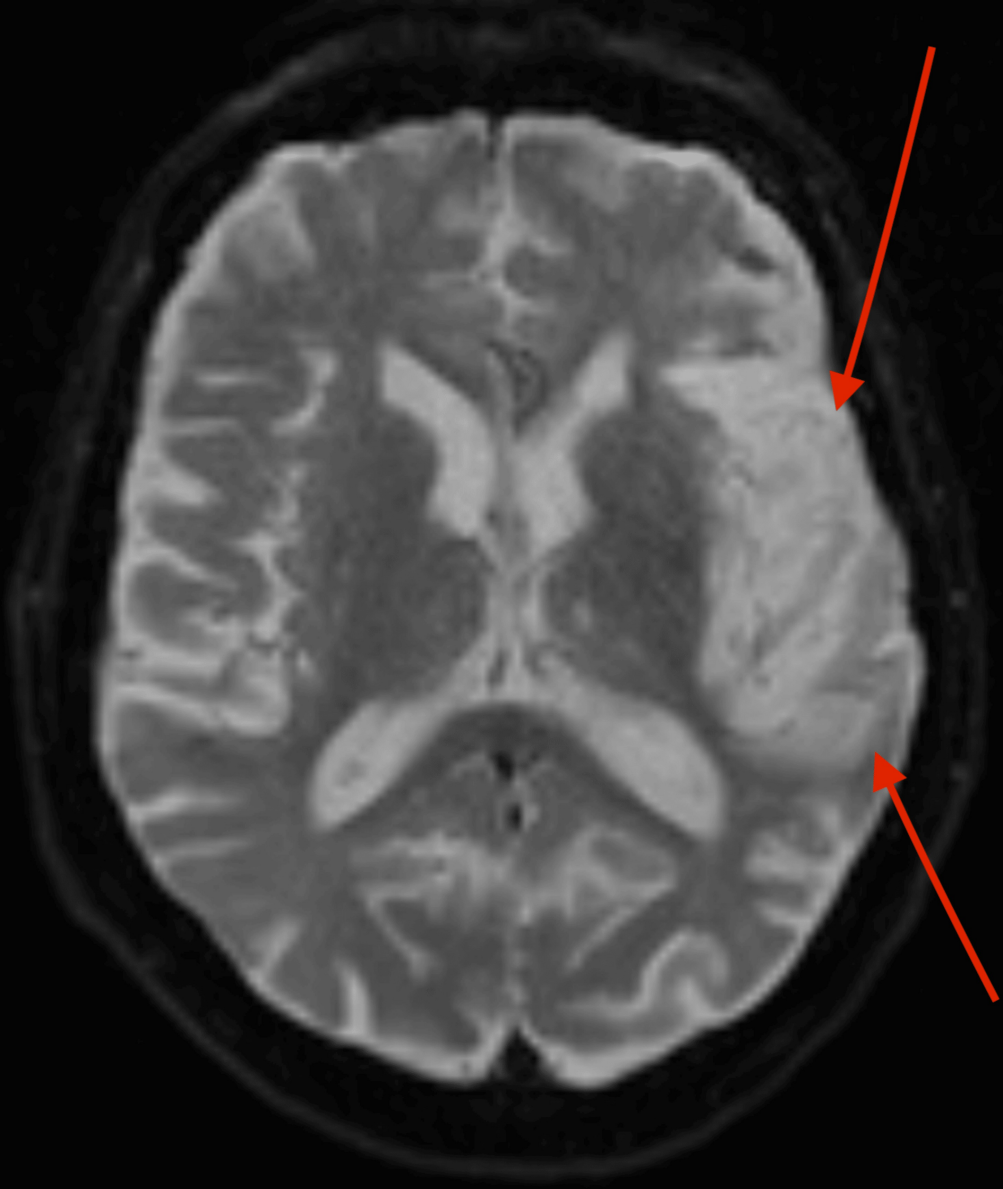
Diffusion-Weighted Magnetic Resonance Imaging (DWI MRI) of the Brain Showing Subacute Infarcts Axial DWI sequence demonstrating large areas of restricted diffusion in the left temporal and frontal lobes (highlighted by arrows), consistent with subacute infarction. These findings, alongside apparent diffusion coefficient (ADC) mapping and other imaging features, supported the diagnosis of cerebral amyloid angiopathy.
